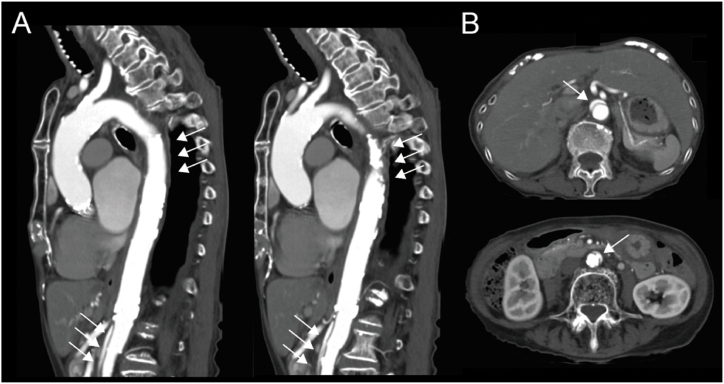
Postoperative computed tomography images of the aorta. (A) Sagittal plane of the aorta. Aortic dissection was observed from the distal arch to the left common iliac artery. (B) Axial plane of the aorta. The false lumen was compressed by the true lumen.
